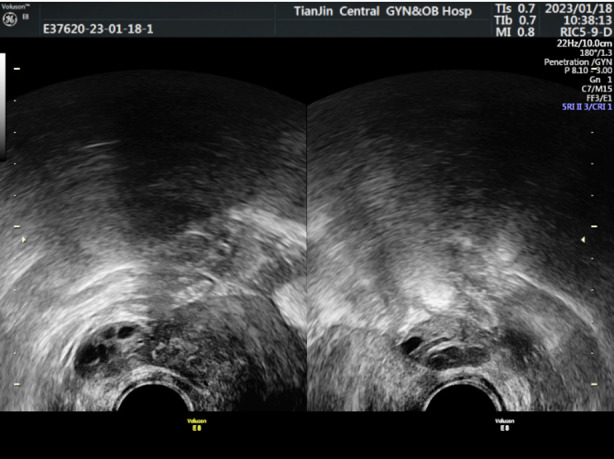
Ultrasonic examination image of DOR-group.
